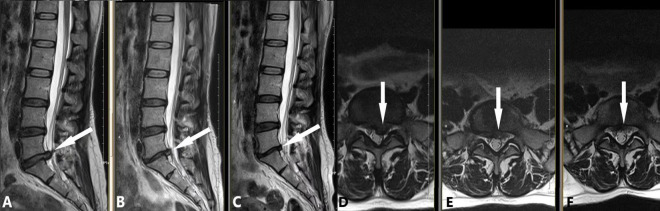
An early chemonucleolysis case demonstrating long-term follow-up and potential complications. A 48-year-old female patient presented with a 2-week history of bilateral sciatica and 1-week history of perianal heaviness. This early case, performed during our initial experience, followed this strategy: (1) Intradiscal injection of 10 mL oxygen-ozone mixture and 200 U collagenase; (2) Extradiscal injection of 1000 U collagenase and 6.5 mL anti-inflammatory analgesic solution. MRI findings are as follows: (A,D) Preoperative images showing L5/S1 posterior disc protrusion (white arrow; A: sagittal view, D: axial view) compressing the dural sac. (B,E) 90-day follow-up images demonstrating significant protrusion resorption (white arrow; B: sagittal view, E: axial view) with L5/S1 intervertebral space narrowing. (C,F) 180-day follow-up images showing further protrusion reduction (white arrow; C: sagittal view, F: axial view), with L5/S1 space narrowing unchanged from the previous exam.
